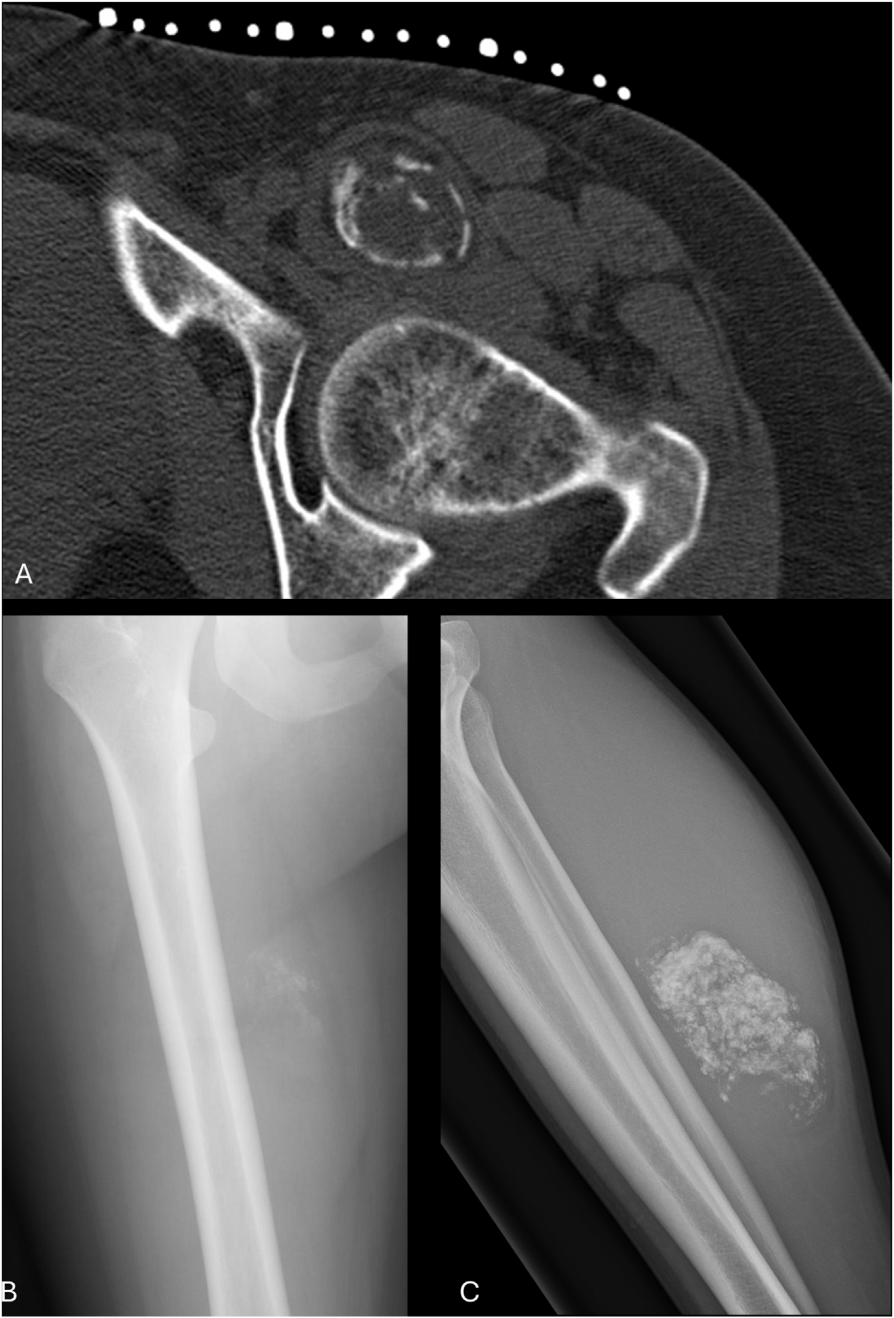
Radiology of calcified soft tissue masses. Axial CT shows an ossifying fibromyxoid tumour anterior to the left hip with a thin, incomplete bone margin (A); AP radiograph of the right femur shows faint amorphous mineralisation projected medial to the bone in a synovial sarcoma (B); lateral radiograph of the right tibia and fibula shows dense mineralisation in the posterior calf in a low-grade fibromyxoid sarcoma (C). Each case shown harboured the relevant recurrent fusion gene characteristic of the tumour type namely PHF1::HCFC1, SS18-SSX and FUS::CREB3L2.
